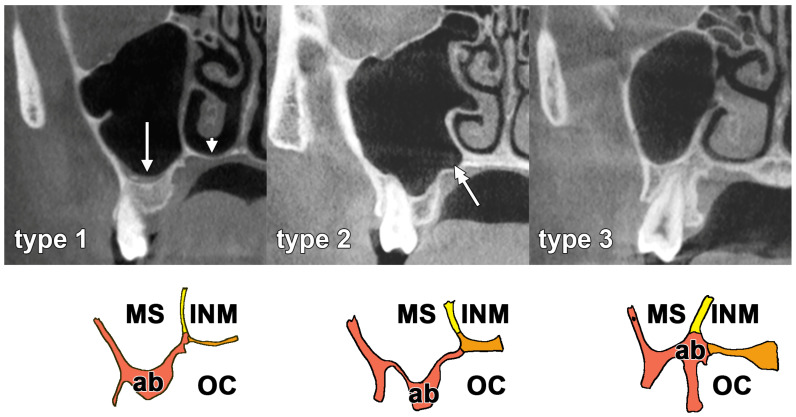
Coronal CBCT slices through the second upper molars depicting the types of pneumatisations above the maxillary alveolar bone. The antral floor (arrow), nasal floor (arrowhead), and palatine recess of the MS (double-headed arrow). Type 1: antral; Type 2: antral, with palatine recess; Type 3: antral and nasal (Big Nose pattern). MS: maxillary sinus; INM: inferior nasal meatus; OC: oral cavity; ab: alveolar bone.
